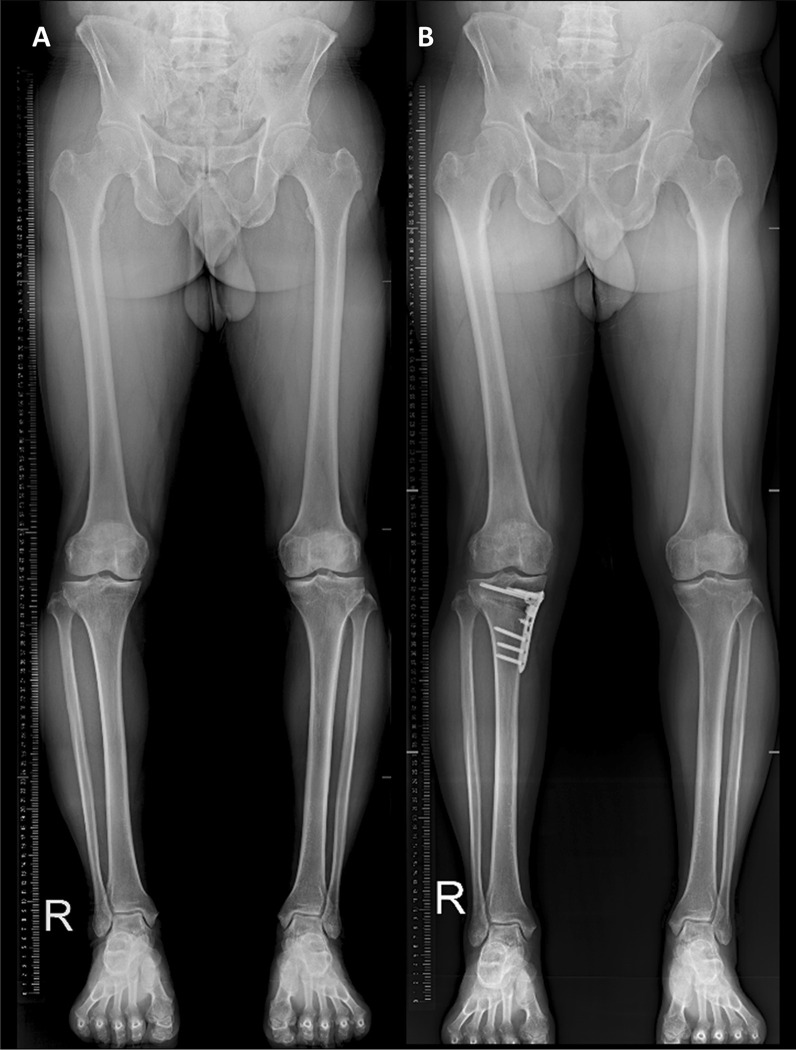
Anterior–posterior standing hip–ankle radiographs of a 55-year-old man. A Preoperative radiograph showing varus limb alignment. B Postoperative radiograph showing correction of varus malalignment and valgus limb alignment after medial open wedge high tibial osteotomy
